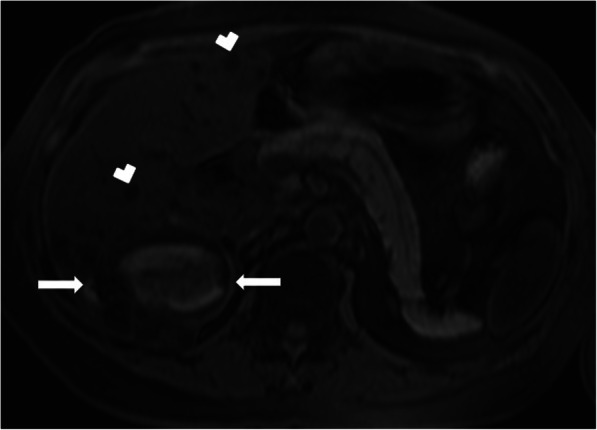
A 44-year-old female patient with known PLD presenting with right upper quadrant pain. On the US, a large hyperechoic mass was found in the right hepatic lobe (not shown). Axial plane precontrast T1W MR image shows subcentimeter cysts (arrowheads) and a cystic lesion with hyperintense content (arrows). There was no discernible enhancement on postcontrast series (not shown). Imaging findings were found to be consistent with the hemorrhagic cyst. Follow-up imaging studies demonstrated the decrease in the size of the lesion
